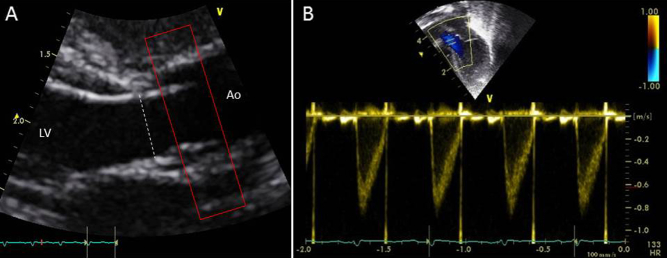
Echocardiographic quantification of LVO, with (A) diameter measured from the parasternal long-axis view and (B) velocity assessed from the apical view. Approximate location of PC MRI acquisition plane is also demonstrated (*red box*). *Ao*, Aorta; *LV*, left ventricle.
